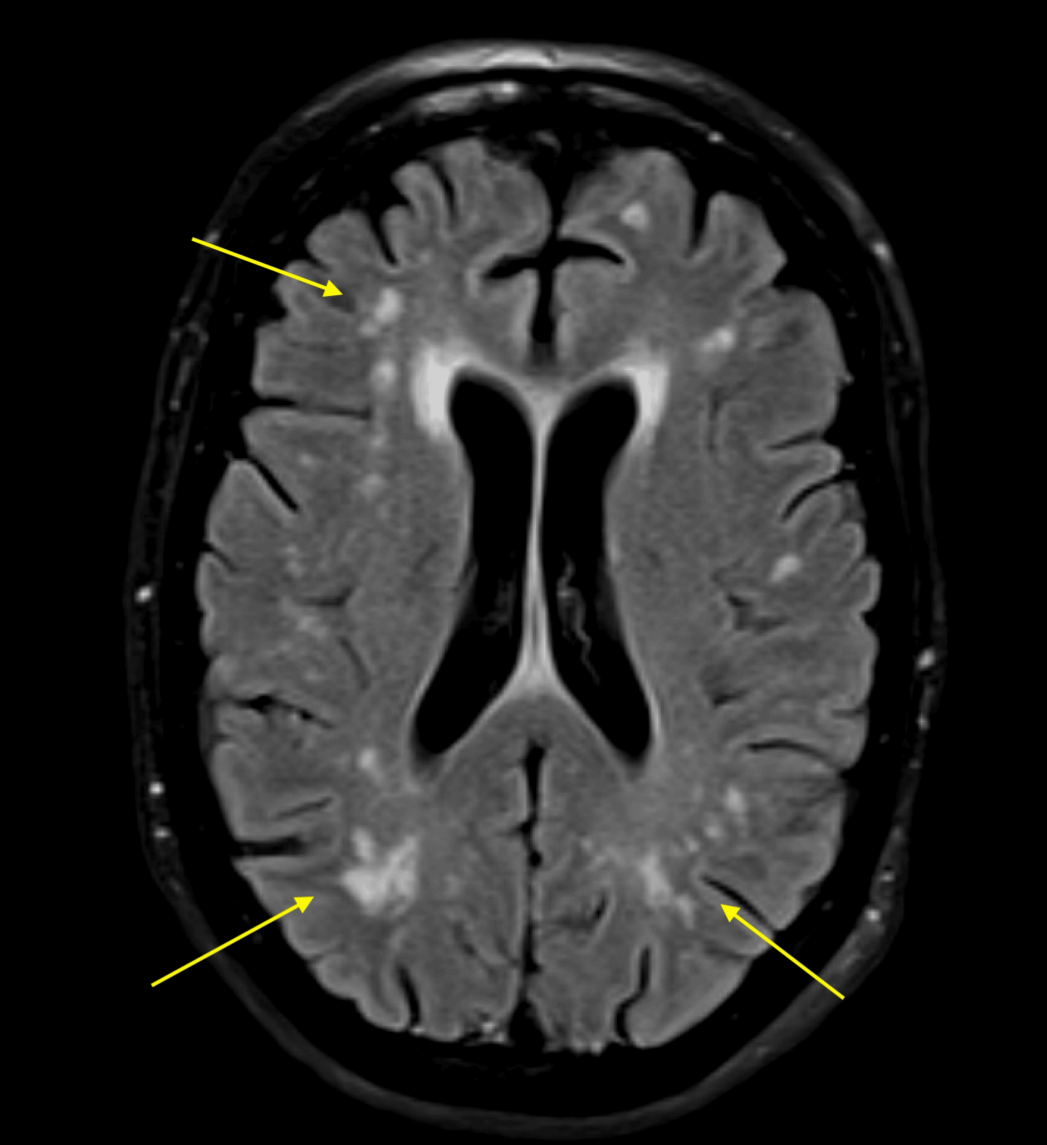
Magnetic resonance imaging of the brain demonstrating patchy supratentorial white matter hyperintensities on fluid attenuated inversion recovery (FLAIR) images
